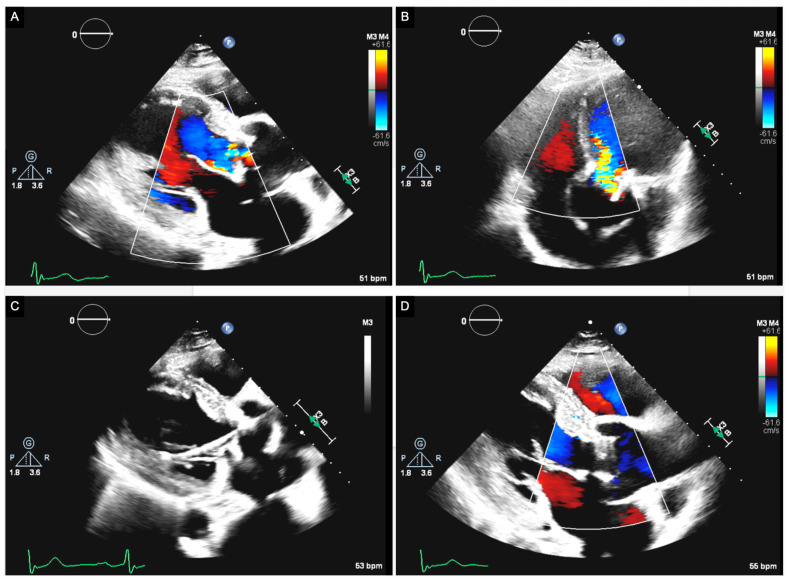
A 77-year-old male with a history of SAVR ten years ago. The patient developed severe SVD, and a valve-in-valve TAVR procedure was indicated. In the preoperative echocardiographic images (Panels A,B), moderate aortic valve stenosis and severe aortic valve regurgitation were observed. A 26 mm Edwards Sapien 3 Ultra transcatheter aortic valve bioprosthesis was implanted via a transfemoral approach (Panel C). Following the procedure (Panel D), a normal aortic prosthesis was observed with normal gradients (14 mmHg) and no prosthetic or periprosthetic aortic valve regurgitation.
